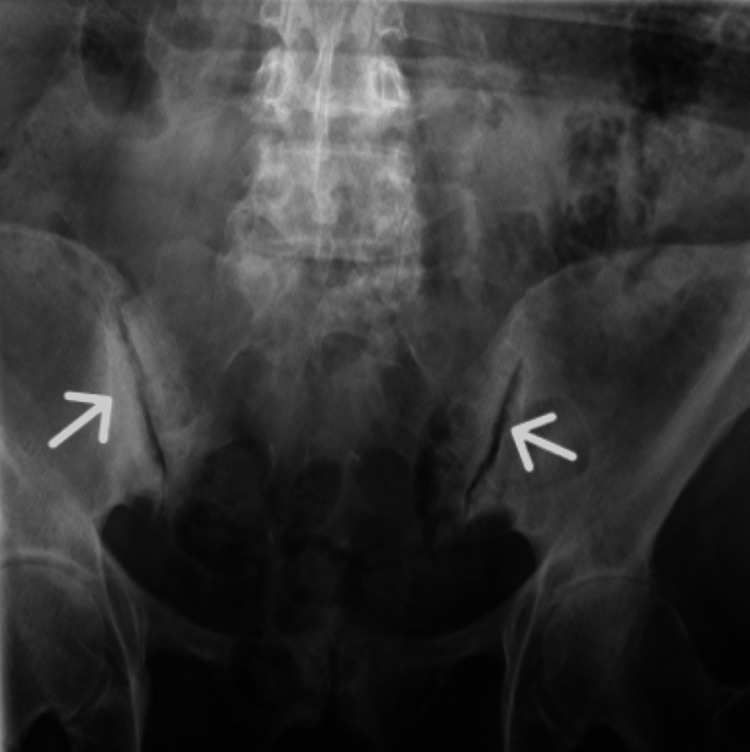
The x-ray image of the sacroiliac joints. The image shows marked bilateral sclerosis around the sacroiliac joints, especially on the iliac bones (arrows) without any evidence of inflammation (such as erosions and narrowing) within the sacroiliac joint spaces (diagnostic of osteitis condensans illi).
